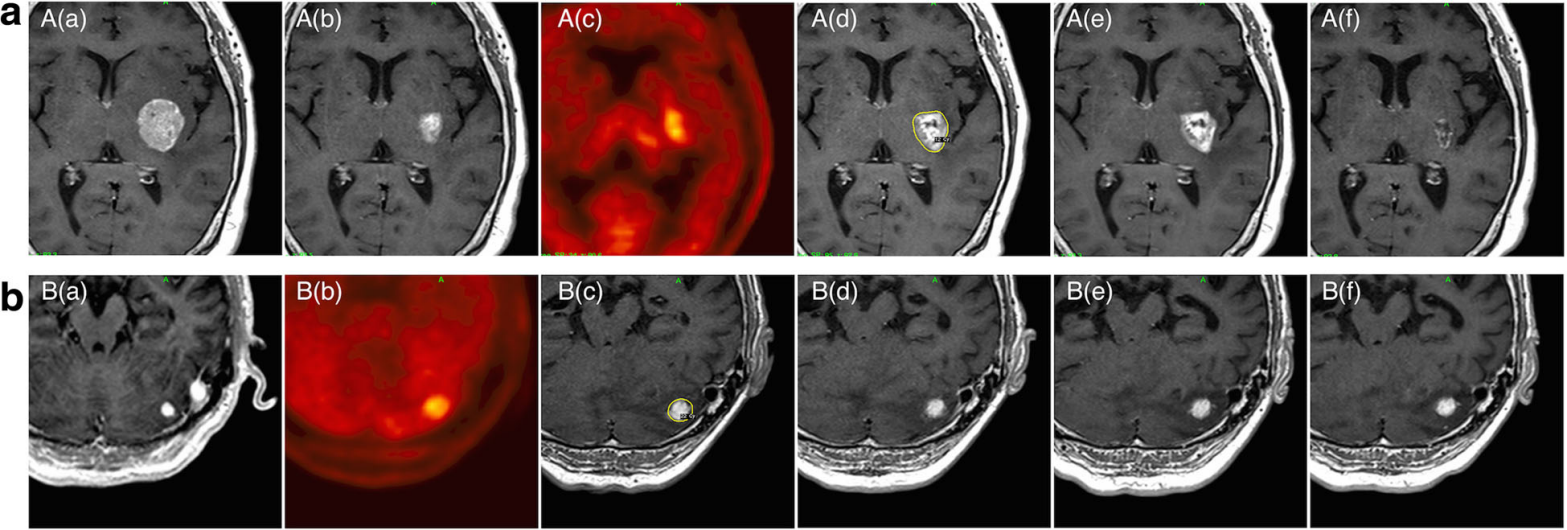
Serial axial Gd-enhanced MR images and MET-PET images of two cases in which MET-PET diagnoses could not be confirmed even with sufficient follow-up after salvage treatment. a: 60s–year-old-woman with multiple brain metastases from breast cancer. (a) Gd-enhanced MR image obtained at the time of the initial SRS. (b) Gd-enhanced MR image obtained three months after SRS. (c) MET-PET image obtained 8 months after initial SRS. The LNR was 1.67. (d) Gd-enhanced MR image obtained at the time of the second SRS. The yellow line represents the prescription isodose volumes (12 Gy at 50%). (e) Gd-enhanced MR image obtained 1 month after the second SRS before BV rescue. Re-irradiation caused neurological worsening and perifocal oedema. (f) Latest follow-up Gd-enhanced MR image obtained 20 months after MET-PET. Repeat BV therapy resulted in symptomatic relief and radiological stabilisation. b: 70s–year-old-man with solitary cerebellar metastasis from gastric cancer. (a) Gd-enhanced MR image obtained at the time of the initial SRS. (b) MET-PET image obtained 19 months after the initial SRS. The LNR was 1.50. (c) Gd-enhanced MR image obtained at the time of the second SRS. The yellow line represents the prescription isodose volumes (22 Gy at 50%). (d) Gd-enhanced MR image obtained 12 months after the second SRS. Re-irradiation caused cerebellar ataxia, requiring temporary conservative treatment with oral steroids. (e) Gd-enhanced MR image obtained 26 months after the second SRS. (f) Latest follow-up Gd-enhanced MR image obtained 36 months after MET-PET. Symptomatic relief and radiological stabilisation were maintained during long-term observation
